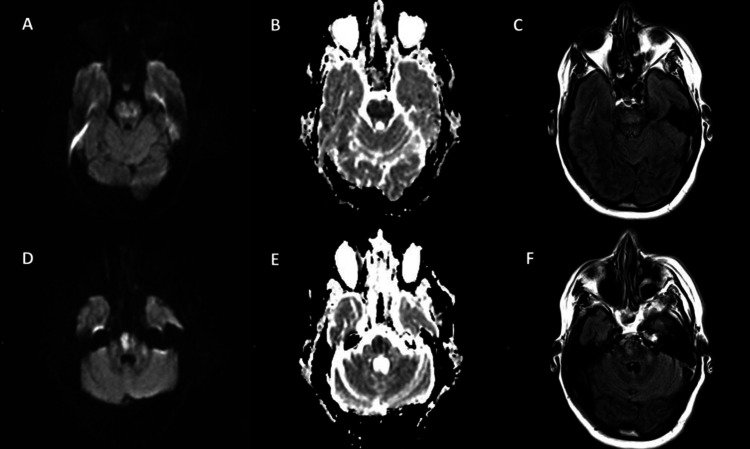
Axial diffusion-weighted imaging (B1000) demonstrating restricted diffusion of the central pons (A and D) with correlate hypointense signal on ADC map (B and E). Correlate T2 FLAIR hyperintense signal (C and F) indicative of cytotoxic edema. ADC: apparent diffusion coefficient, FLAIR: fluid-attenuated inversion recovery
